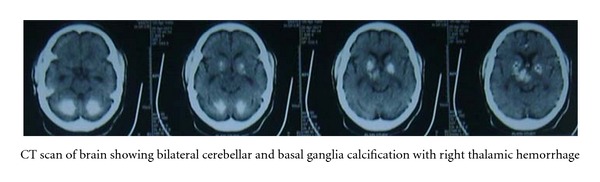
Plain CT scan of head showed bilateral basal ganglia and cerebellar calcification with right thalamic and midbrain hemorrhage without any midline shift.
